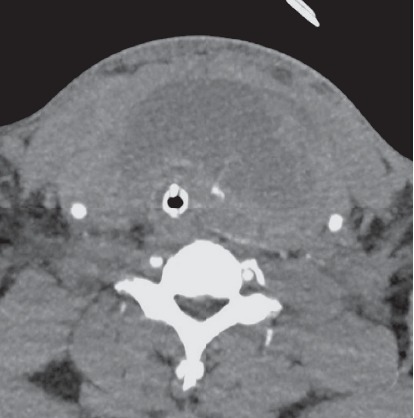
Axial CTA scan demonstrating thyroid hematoma and collapsed trachea with arterial blush concerning for active hemorrhage in the thyroid parenchyma.
